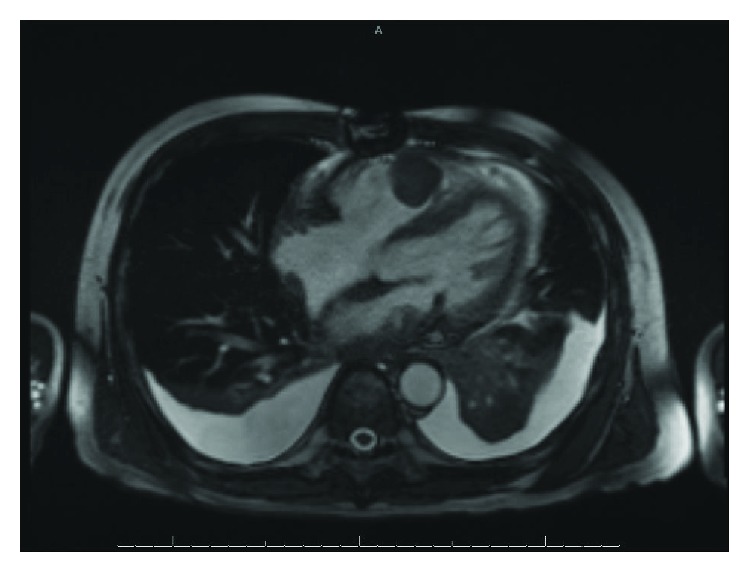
MRI confirmed masses in the right and left ventricle. Shown above is an image of the mass located in the right ventricle.
